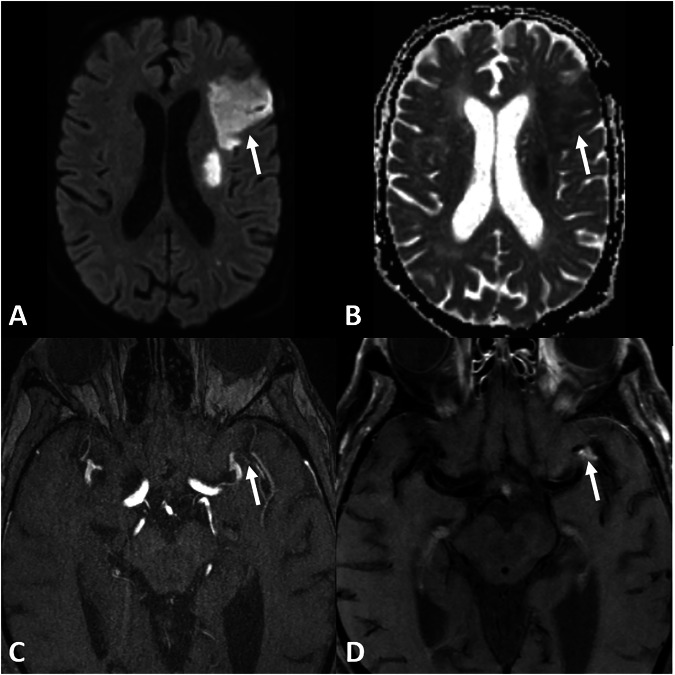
Follow-up imaging of the same 74-year-old male patient as Fig. 1, 13 days later, despite the initiation of immunosuppressant therapy. There is a new infarction on the DWI B1000 (arrow) (A) with decreased ADC values (arrow) (B) in the left MCA territory. The MCA stenosis remains constant (arrow) (C), whereas the vessel wall enhancement is slightly regressing (arrow) on T1 TSE dark blood (D)
